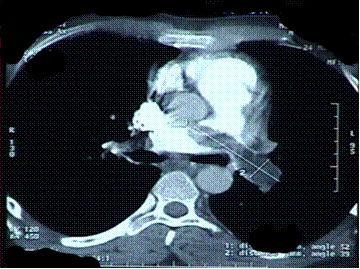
High-resolution computed tomographic image of the patient's chest showing the saddle embolus.
